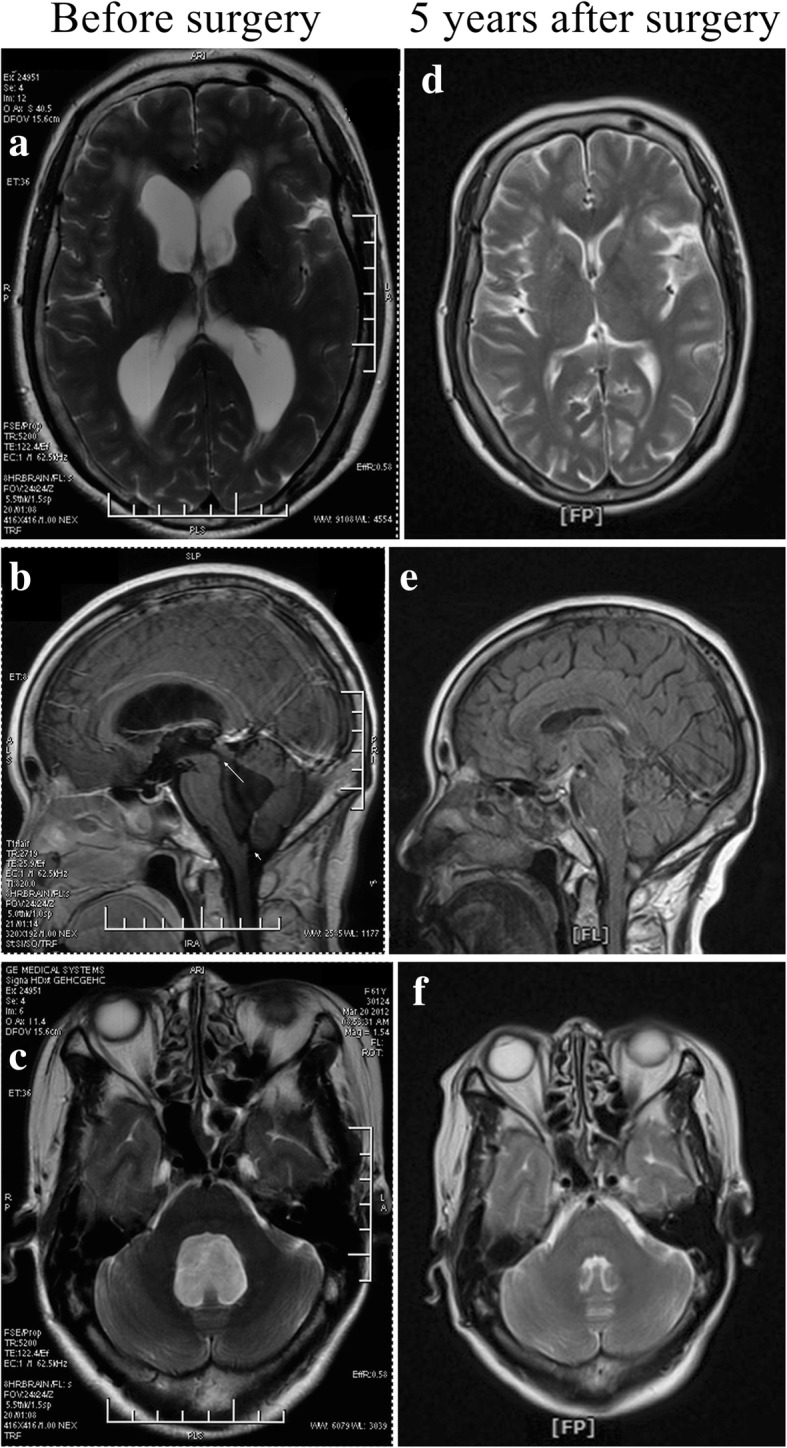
Preoperative (a–c) and postoperative (d–f) magnetic resonance imaging of a typical disproportionately large communicating fourth ventricle in patient 1. → Preoperative dilated aqueduct
